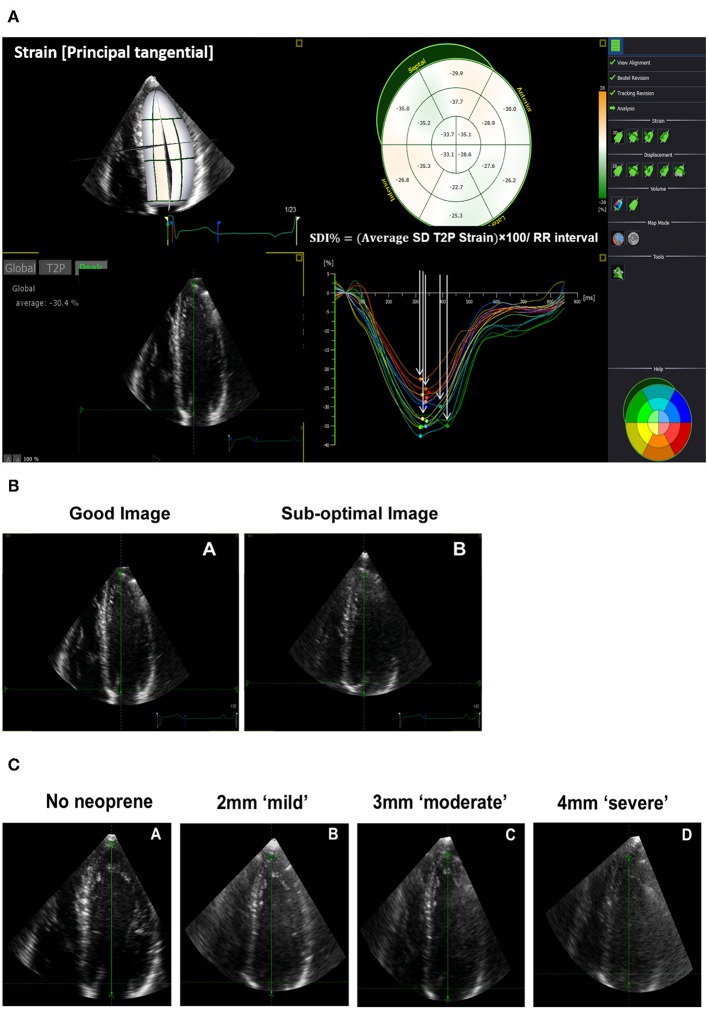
Three-dimensional echocardiographic (3DE) strain derived systolic dyssynchrony index (SDI) and impaired quality 3DE images. (A) SDI derived from principle tangential strain. (B) An example of a good and sub-optimal 3DE image quality obtained from the same participant in study 1. (C) An example of a 3DE with an optimal quality reference (no neoprene), mild (2 mm neoprene), moderate (3 mm neoprene), and severe (4 mm neoprene) impairment of 3DE image quality obtained from the same participant in study 2.
